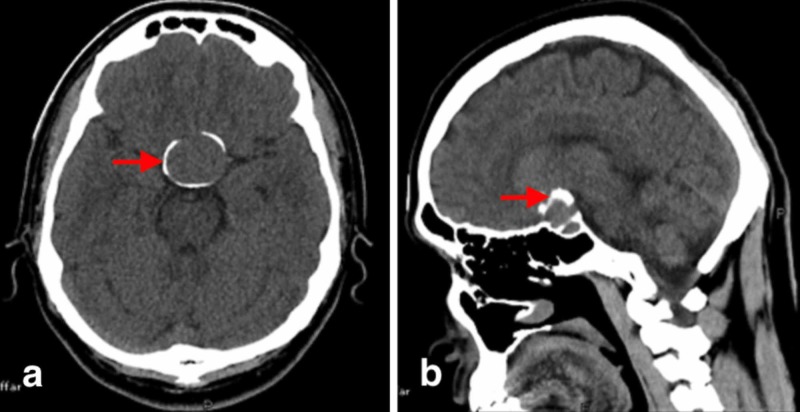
(a) CT scan brain plain, axial section showing round, compact calcification around sellar and suprasellar lesion. (b) CT scan brain sagittal section showing round, compact calcification around sellar and suprasellar lesion.
